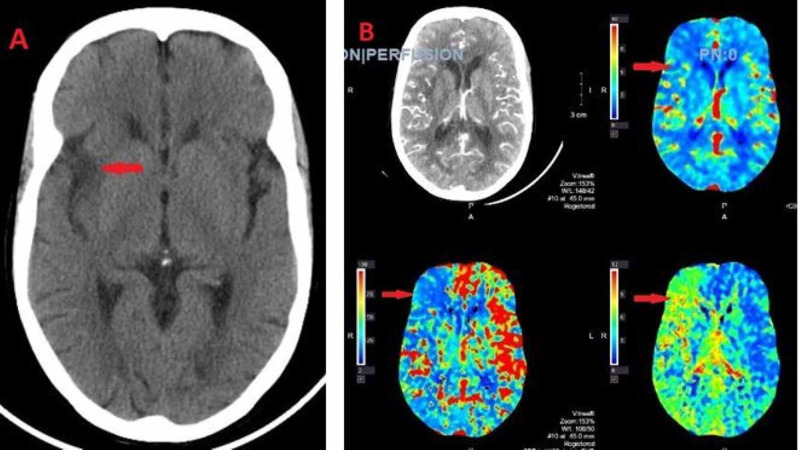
(A) Computed tomography (CT) head with no contrast showing lucency with loss of gray-white differentiation along the right insular cortex and subinsular region compatible with an acute infarct. (B) CT angiogram of the head showing perfusion defect in the right insular cortex and right frontal lobe suggesting a completed core infarct.
